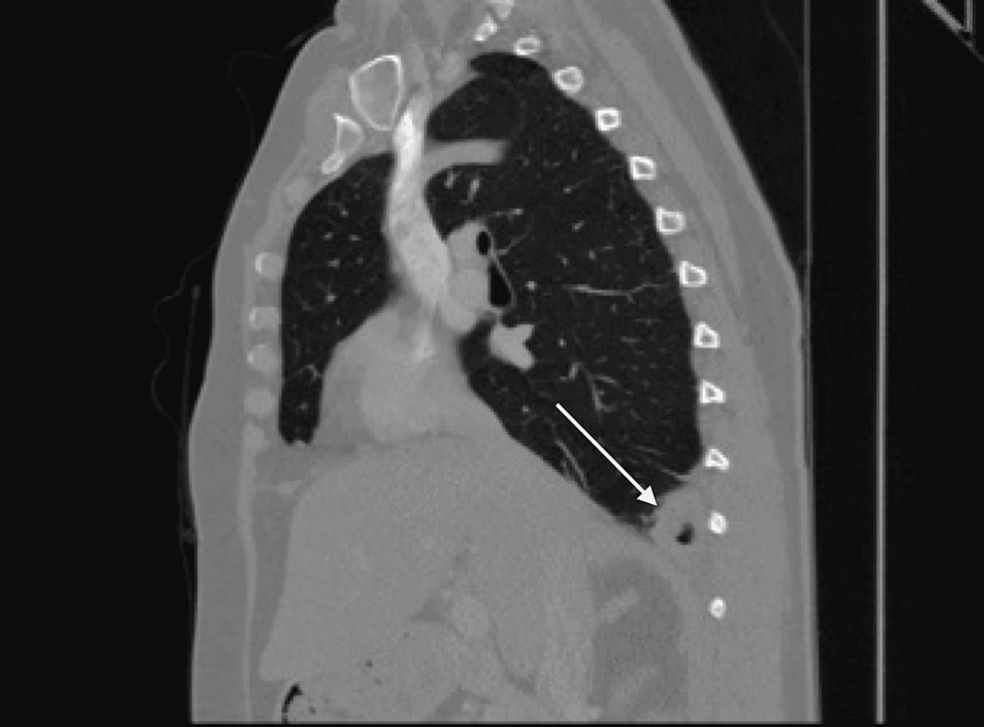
Chest CT (sagittal view) demonstrating right basilar cavitary lesion.
